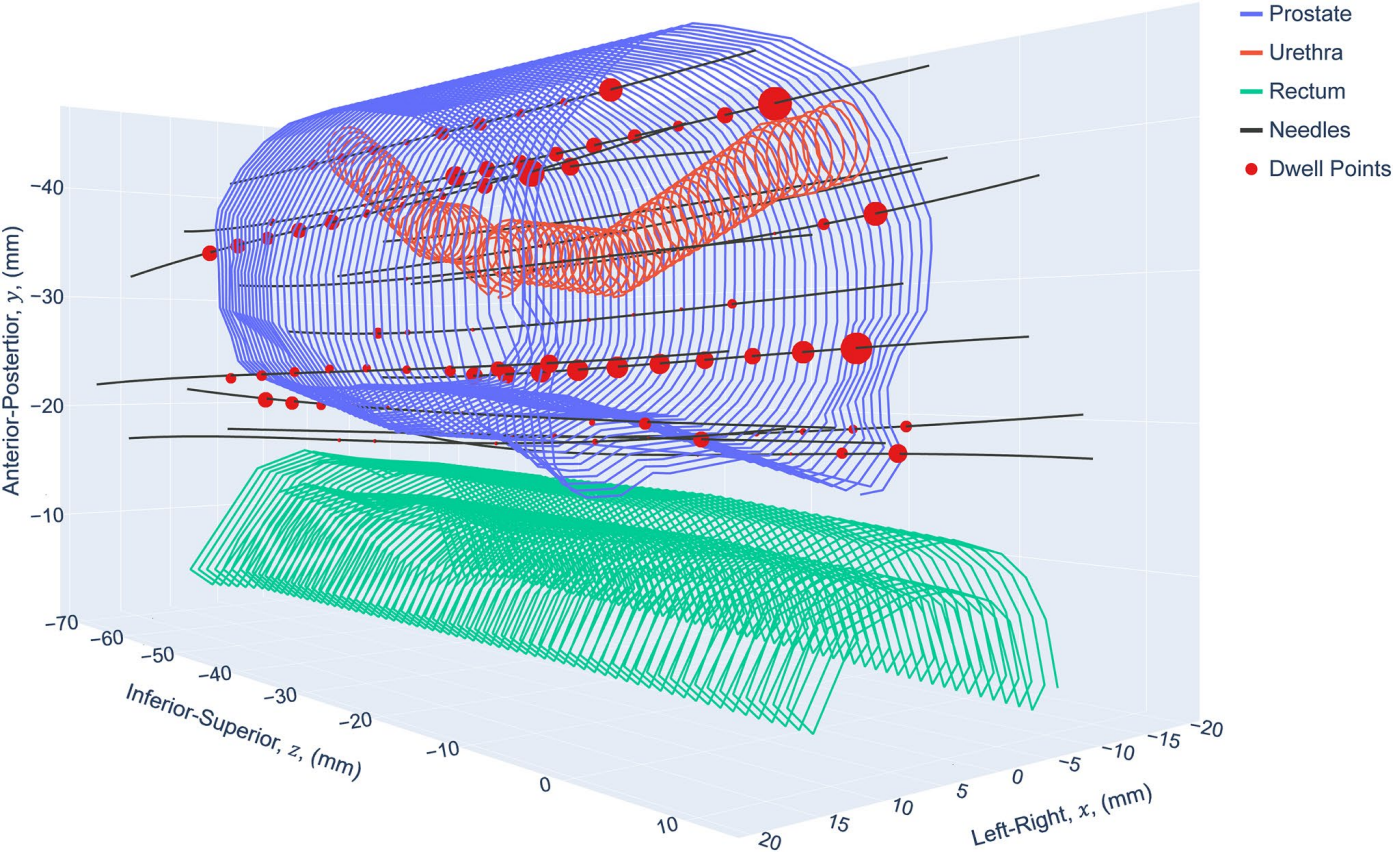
Patient geometry from the HDR prostate brachytherapy ultrasound treatment plan. The size of each red dwell point is directly proportionate to the relative dwell time for the source at that dwell point
